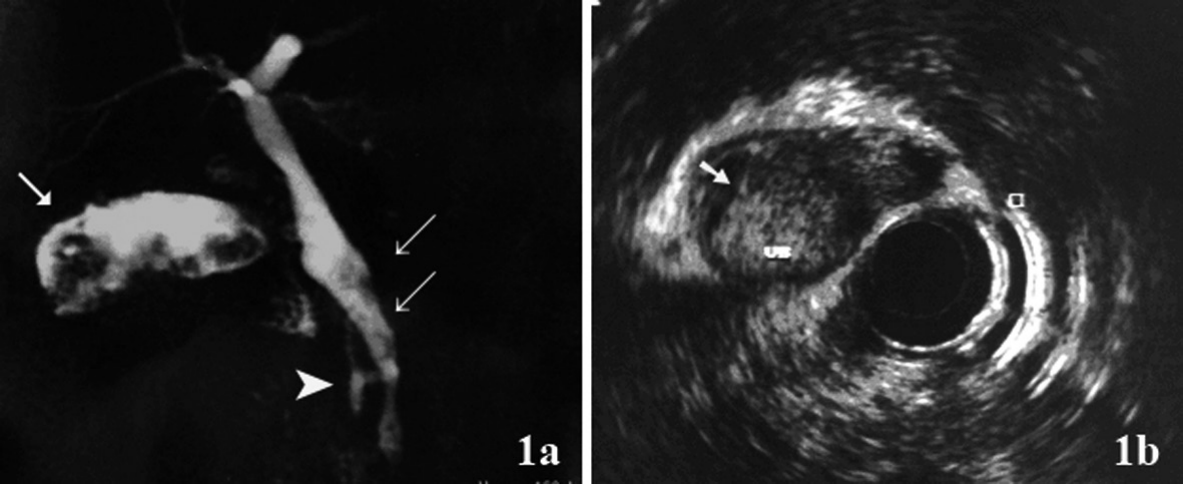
(a) Magnetic resonance cholangiopancreatography (MRCP) showed hyperdense structure within the gallbladder infundibulum, which were suggestive of tumor (arrow), bile duct dilatation and filling defect (double arrow) and pancreaticobiliary maljunction(arrow head); (b) Endoscopic ultrasound showed hyperechoic frond-like mass within the gallbladder (arrow).
